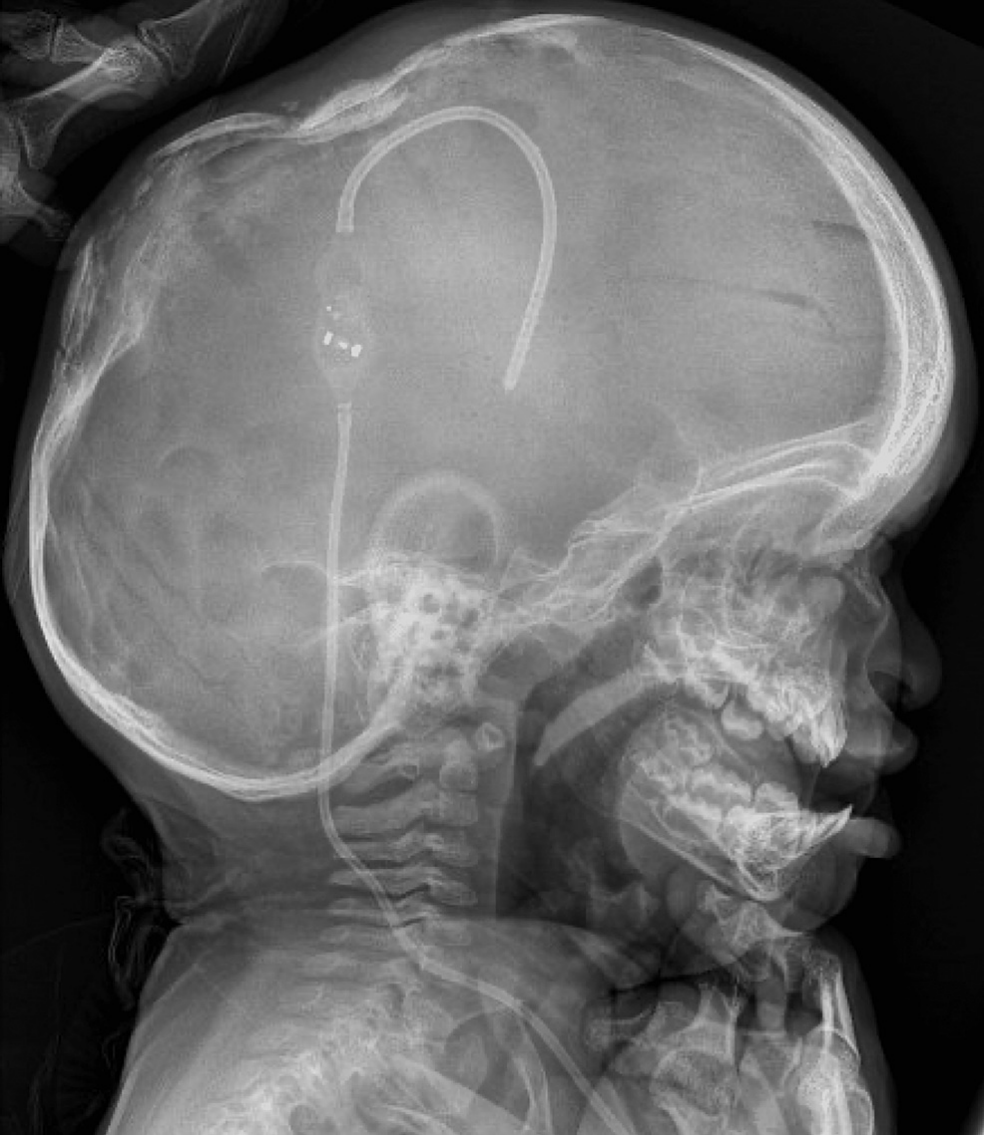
Skull X-ray showing the insertion of a programmable subdural-peritoneal shunt.
